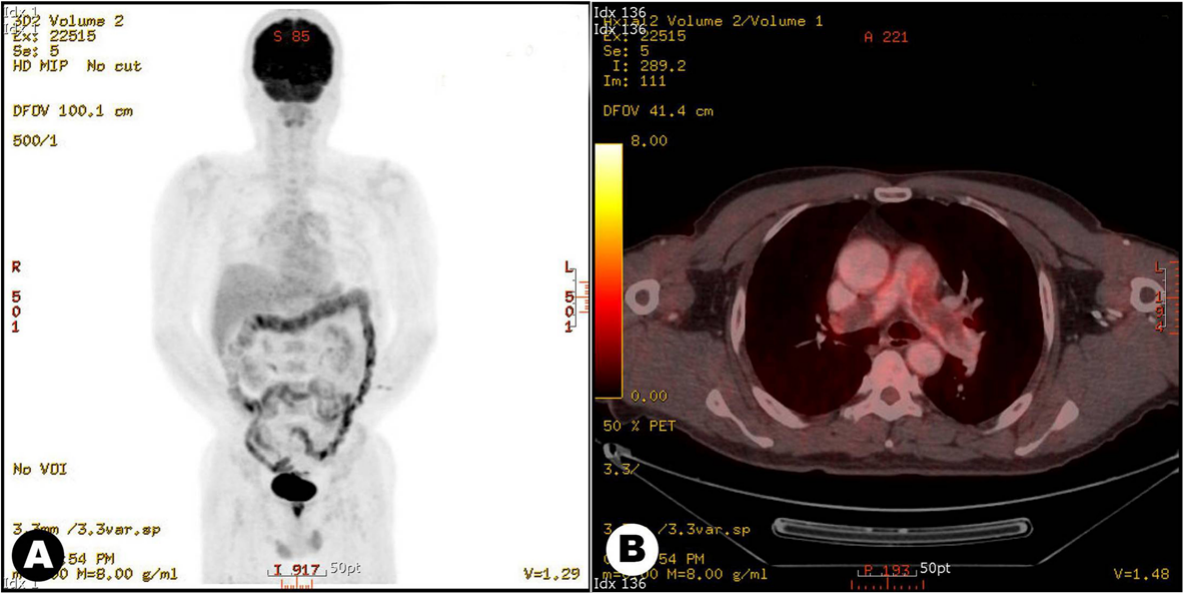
Maximum intensity projection image of ^18^fluorodeoxyglucose positron emission tomography (FDG-PET) shows no specific uptake of FDG in a chest image (left). An axial section shows large intraluminal masses in the pulmonary artery without significant FDG-uptake.
